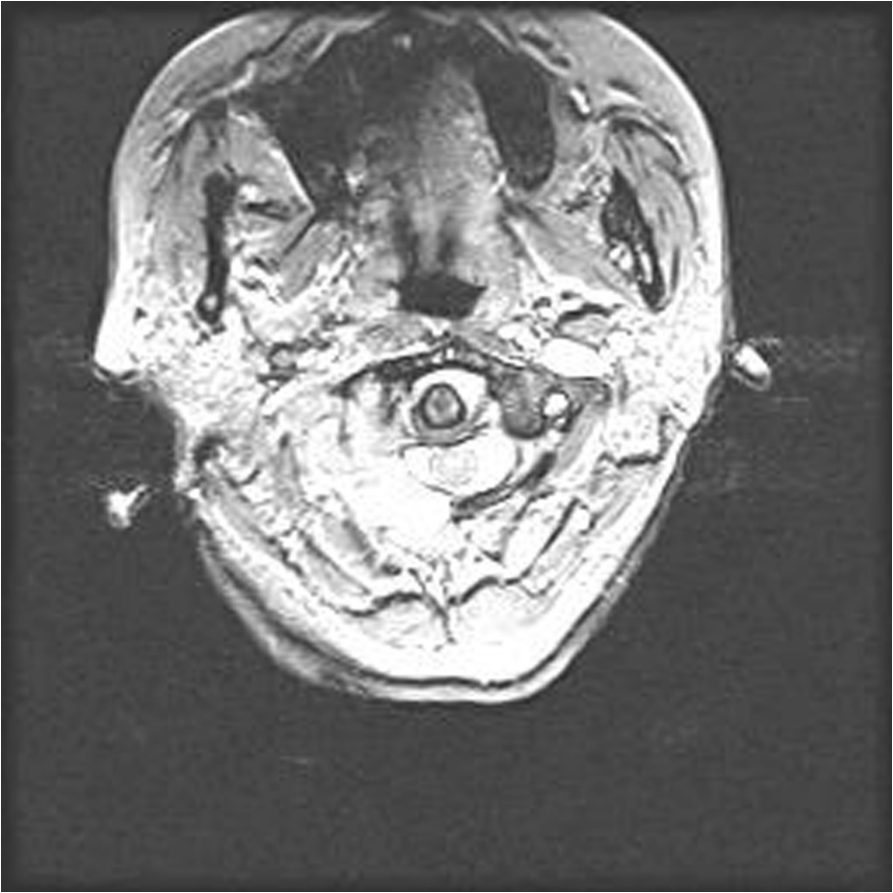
Axial MRI preoperative image.
